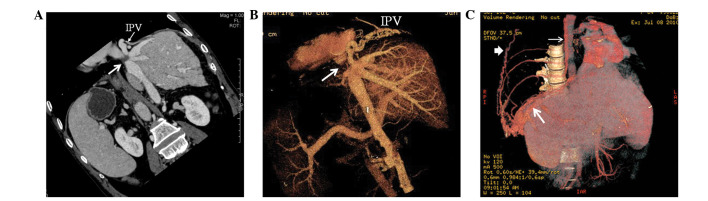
HV-IPV collaterals. (A) CTA shows segmental occlusions of both the IVC and HVs (thick arrow). The dilated IPV can also be seen (thin arrow). (B) Blood in the occluded HVs flows into the IPV. (C) CTA in another patient shows that blood in the occluded HVs flows retrogradely through the web-like IPV (thick arrow), the intercostal veins and then into the azygos vein (thin arrow). Blood from the IPV is also drained by a varicosity over the chest wall in this case (arrow head). HV, hepatic vein; CTA, computed tomography angiography; IVC, inferior vena cava; IPV, inferior phrenic vein.
